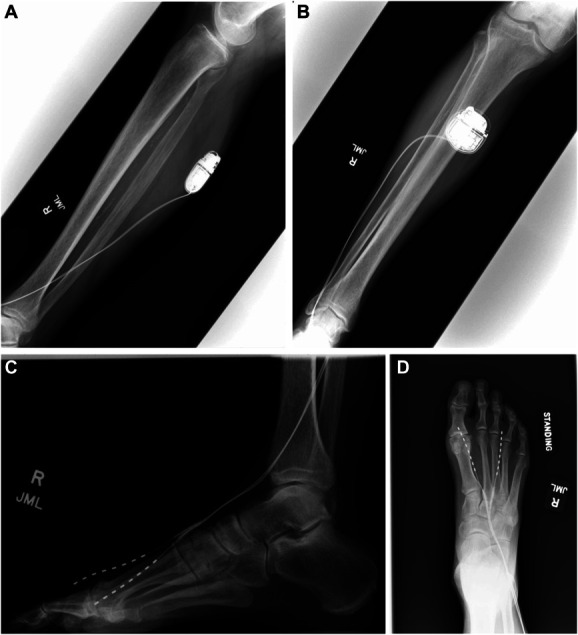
A-D, Postoperative x-rays of the patient's lower extremity demonstrating positioning of the lead and implantable pulse generator as well as the path of the tunneled lead.
